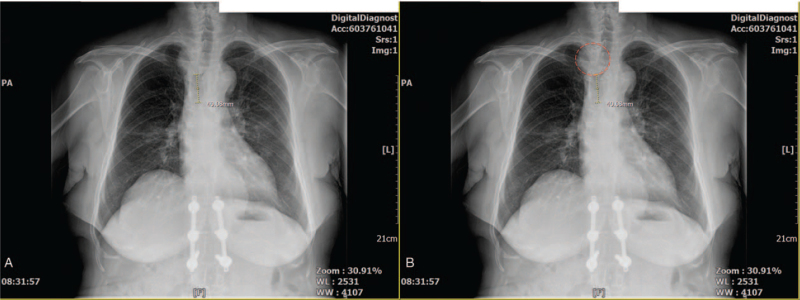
Preoperative posteroanterior chest X-ray showing a tracheal mass compressing the airway 4 cm above the carina (red-dotted circle). To facilitate the identification of the mass that is not clearly visible (A), the red-dashed circle surrounding the mass was placed in the image (B).
